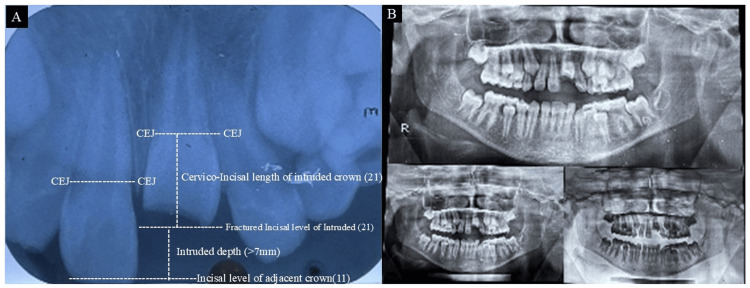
Preoperative radiographic assessment. A) Intraoral periapical radiograph demonstrating complete intrusion of the maxillary left central incisor, with apical displacement, narrowing of the periodontal ligament space, and a closed apex. No root or alveolar bone fractures are evident. B) Panoramic radiograph confirming the extent of intrusion and the absence of associated dentoalveolar fractures.
